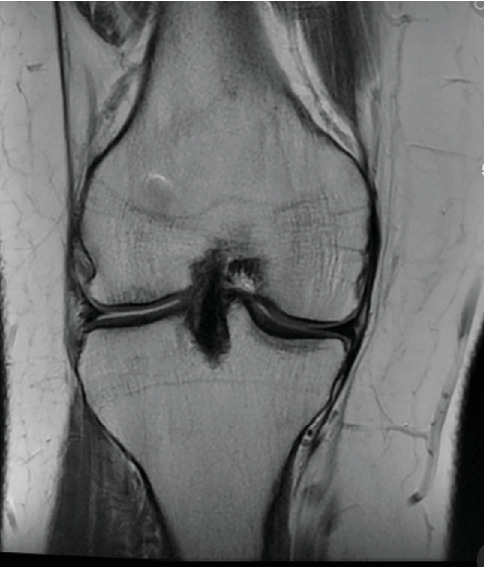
MRI from 09/2022 again demonstrating fill of the lateral tibial plateau cartilage defect.
